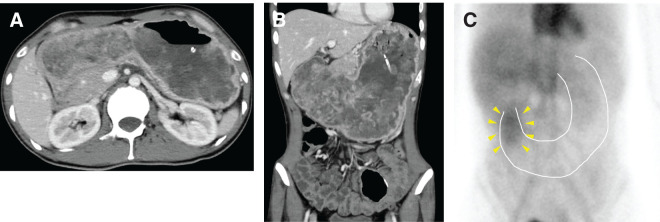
CT findings in axial (A) and coronal (B) views. The stomach is significantly enlarged, and numerous polyps are observed inside. (C) Technetium-99m human serum albumin scintigraphy demonstrated increased tracer accumulation in the gastric antrum 6 h after injection. White dotted lines: gastric outline; yellow arrowheads, tracer accumulation.
